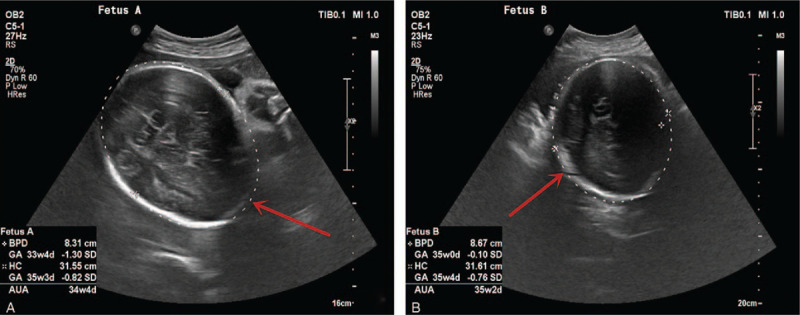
(A and B) Ultrasound scan images showing the fetuses. (A) is the first fetus, whereas (B) is the second fetus. Red arrows.
